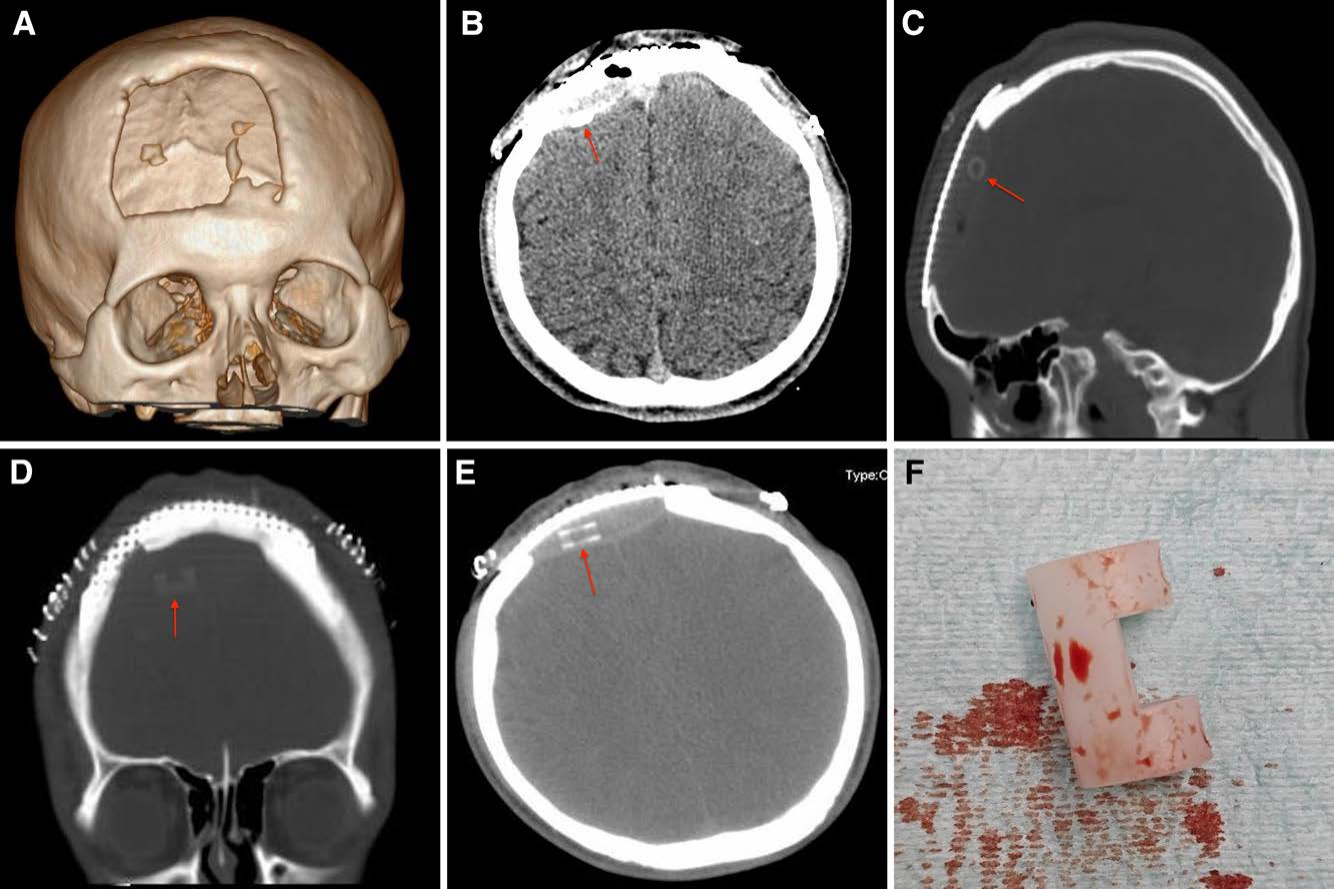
(A–F) are images of an 18-yr-old male patient underwent surgery and a Raney clip was left behind the titanium mesh after the surgical procedure. (A) Show the bone window via which the abscess was evaluated in the first operation. (B) CT scan showing an opacity that was mistaken for epidural hematoma (Red arrow). Blood cultures did not yield any organisms. (C–E) Are thin-slice CT scan images of the skull showing a high-density shadow beneath the titanium mesh resembling a Raney clip (Red arrows). (F) Is an image of the Raney clip retrieved underneath the titanium mesh. The patient was discharged home 7 d later and 1-yr follow-up revealed the patient is well and go about his daily duties. This case highlights the importance of utmost care and diligence during and after neurosurgical procedures. CT = computed tomography.
